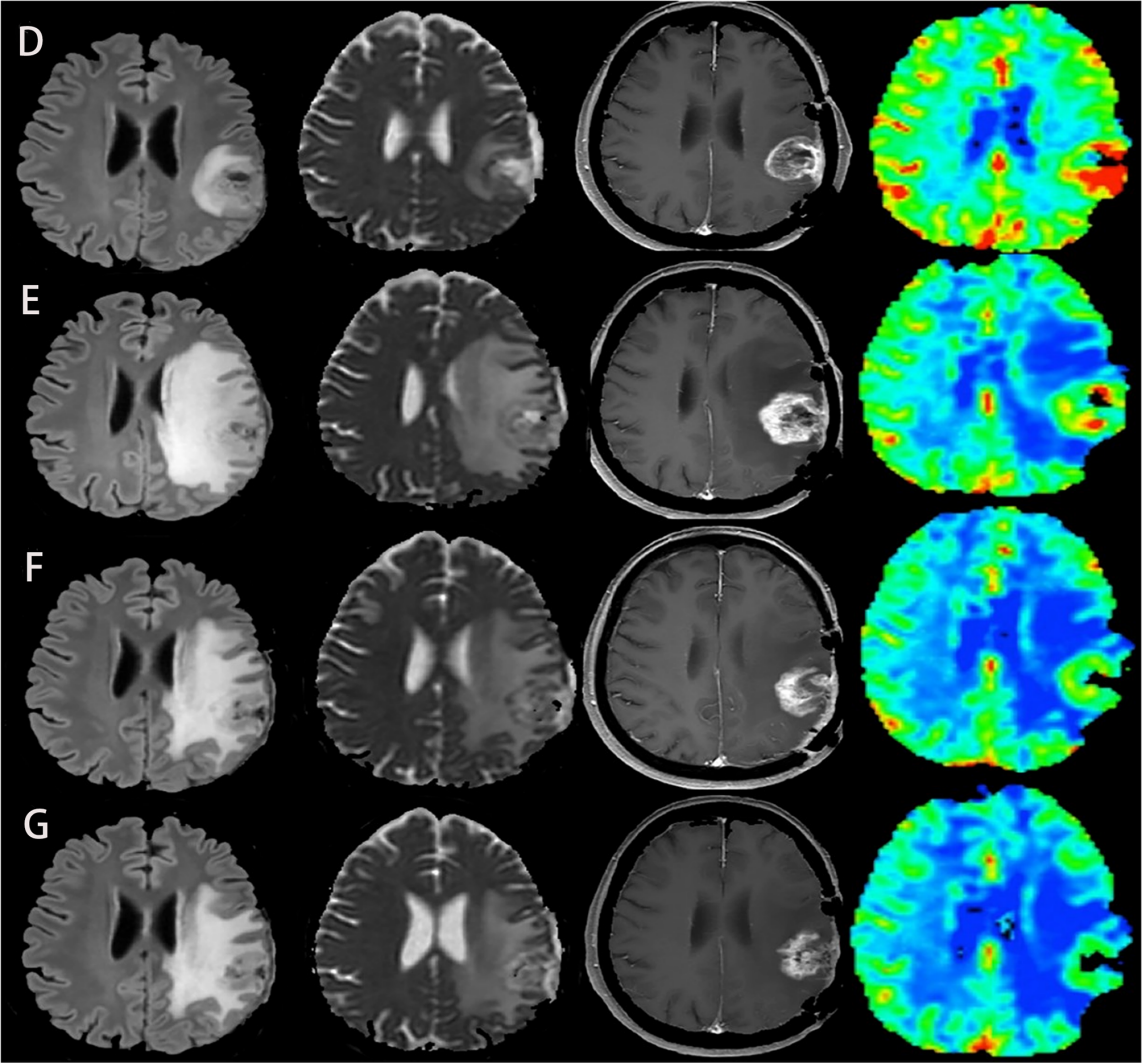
Another case of glioblastoma developed pseudoprogression during immunotherapy, with FLAIR, ADC map, T1-enhanced, and CBV map from left to right. (D–G) MRI was performed before immunotherapy and 2, 4, and 6 months after immunotherapy, respectively. Although tumor recurrence was suspected at the second month, the subsequent two MRI showed that the lesion became smaller, the degree of edema and imitation of diffusion alleviated, and the perfusion decreased. These two cases demonstrate that the combination of conventional MRI and advanced MRI imaging can accurately identify pseudoprogression and tumor recurrence of glioblastoma after immunotherapy. The above two figures were reproduced with the permission of (29) (Copyright at Multidisciplinary Digital Publishing Institute).
